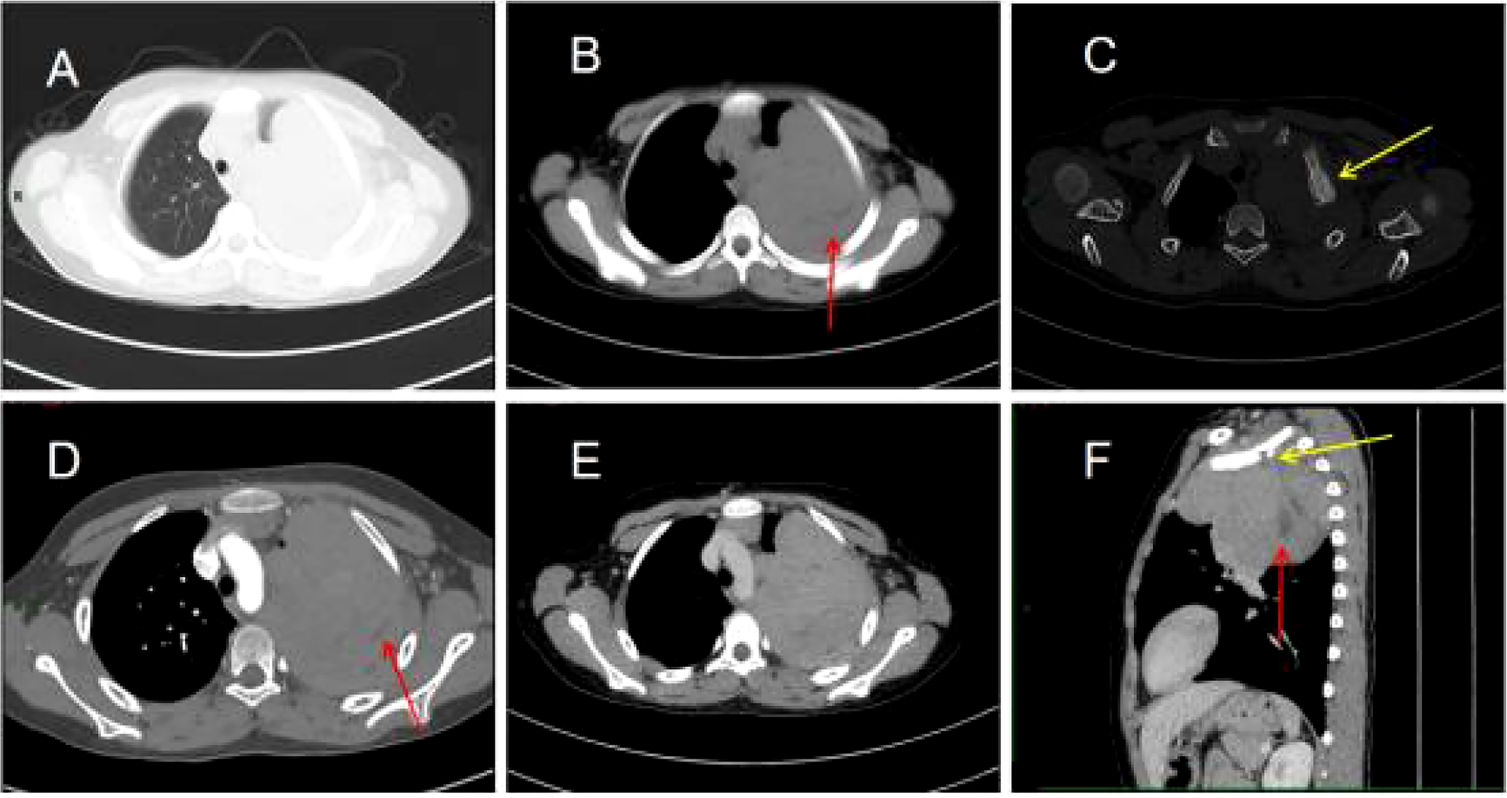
Chest computed tomography (CT) images (A–F). (A) Irregular soft tissue density mass in the upper left thorax. (B) The mediastinal window shows a lamellar hypodense shadow within the mass (arrow). (C) The bone window shows bone destruction of the left first rib and visible periosteal new bone formation (arrow). (D) Mild to moderate heterogeneous enhancement of the lesion with non-enhancing hypodense areas within it (arrow). (E) Persistent enhancement of the lesion in the venous phase. (F) The sagittal section shows bone destruction of the left first rib (yellow arrow) and lamellar hypodense areas within the mass (red arrow).
